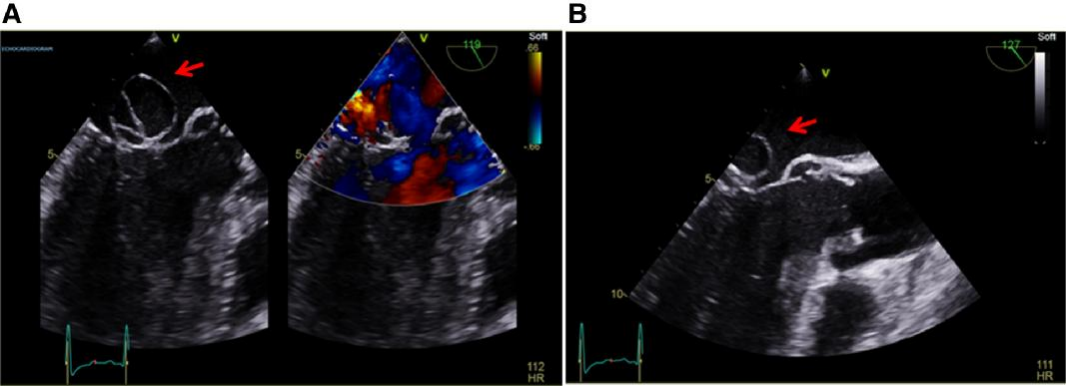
A long-axis trans-oesophageal echocardiogram showing the anterior mitral leaflet aneurysm (see the arrows) and eccentric Doppler jet.
